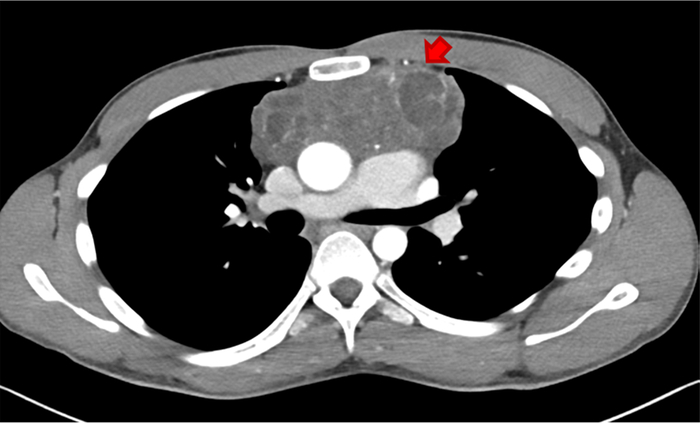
Contrast-enhanced chest CT demonstrating an inhomogeneous soft-tissue density mass (*red arrow*) located in anterior mediastinum. This finding is referable to recurrence of teratoma.
